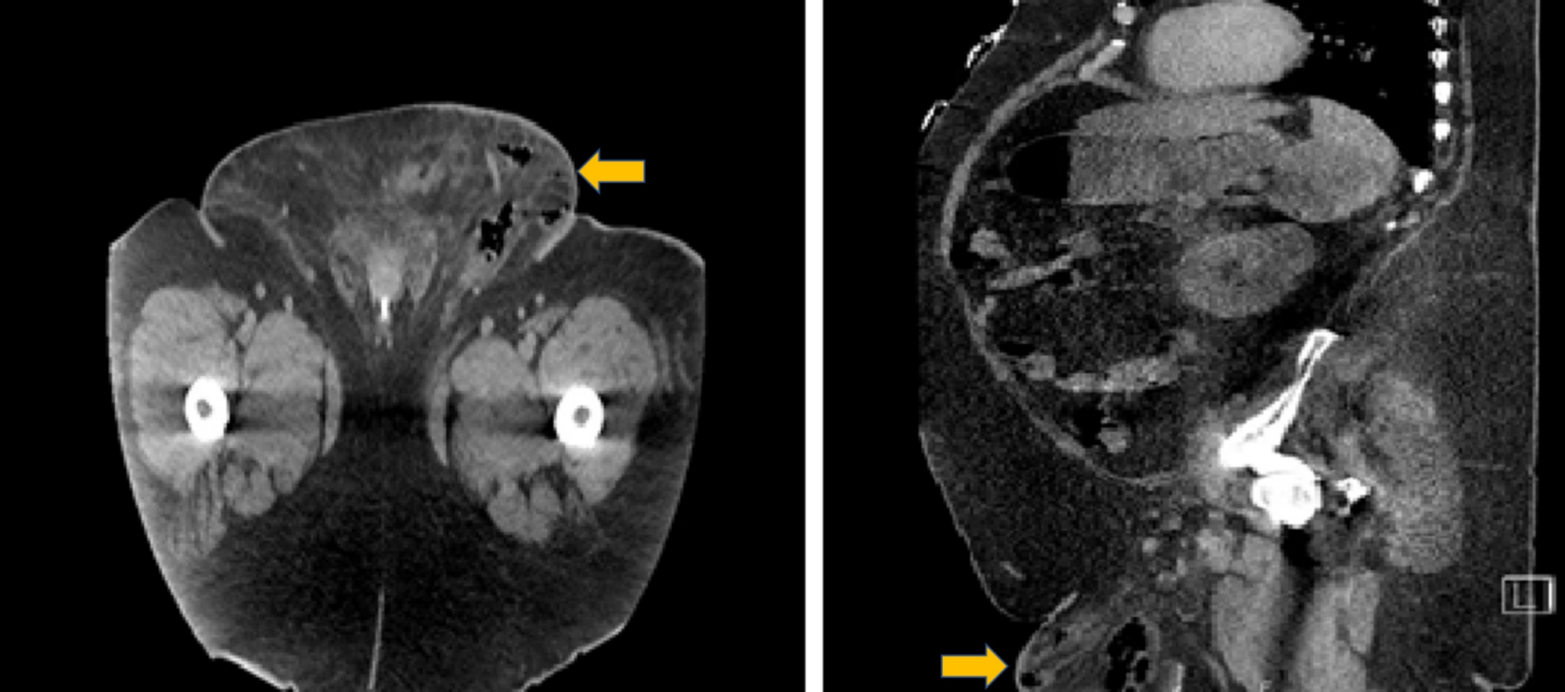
Abdominal CT showing gas in the subcutaneous tissue of the scrotum confirming the diagnosis of Fournier’s gangrene.
